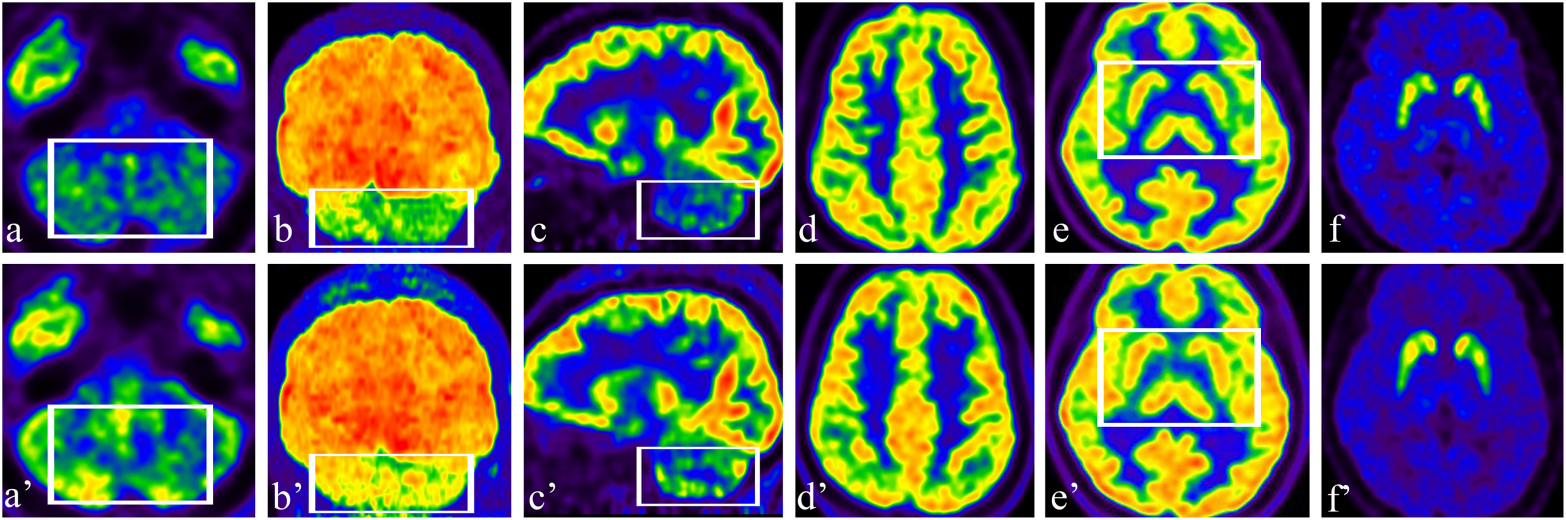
(a–c) Preoperative axial, coronal, and sagittal ^18^F-FDG images showed hypometabolism in the bilateral cerebellar hemisphere; (a'–c') Postoperative axial, coronal, and sagittal ^18^F-FDG images showed increased uptake in the cerebellum (see box). (d, e) Preoperative axial ^18^F-FDG images showed normal metabolism in the bilateral cerebral cortex, GPi area, and basal ganglia; (d', e') There was no change in ^18^F-FDG in the cerebral cortex, GPi area, or basal ganglia after stimulation (see box). (f) Preoperative axial DAT PET/CT showed normal DAT density in the bilateral caudate nucleus and putamen; (f') Compared with the findings in a pre-stimulation image, there were no significant differences in DAT binding in the bilateral caudate nucleus and putamen.
